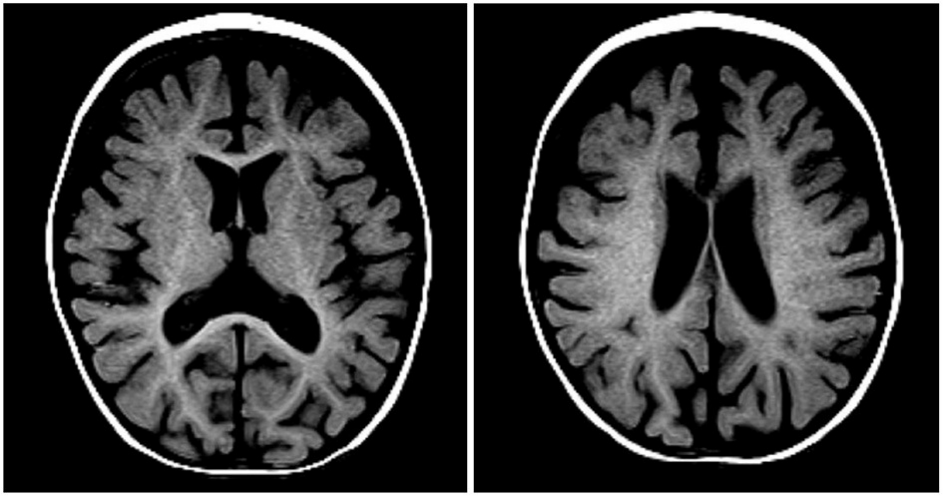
Axial T1 MRI images showing reduced white matter volume resulting in enlargement of the CSF spaces with age-appropriate myelination pattern.
